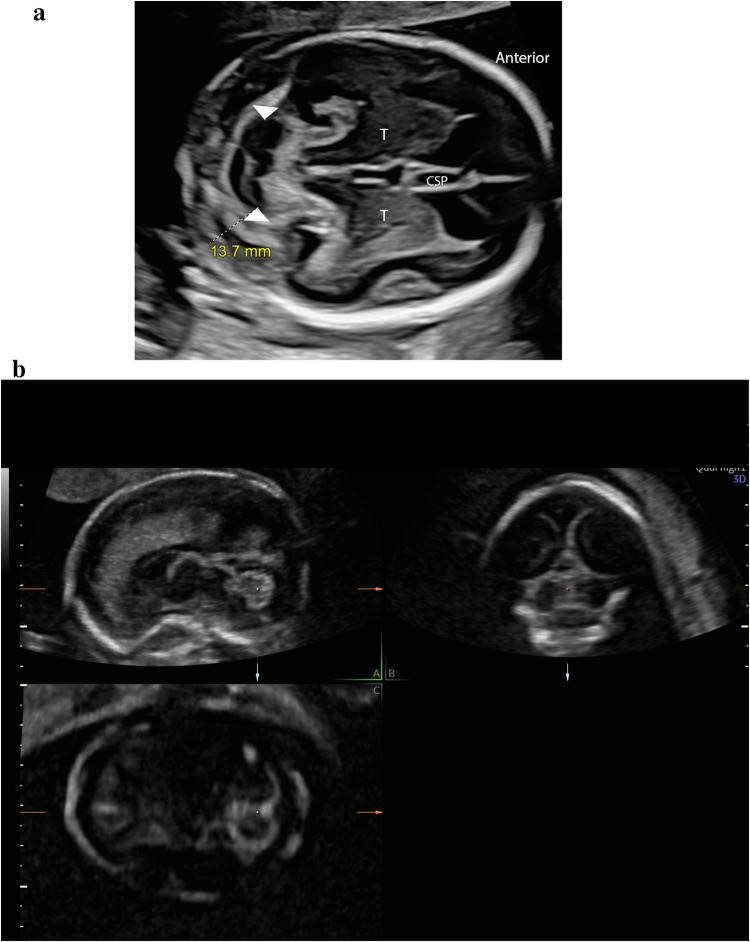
Ultrasound assessment of the posterior fossa in a fetus at 19 4/7 weeks. a Suboccipitobregmatic transverse view of the fetal head aligns the lower cavum septum pellucidum (CSP), thalamic nuclei (T), and cerebellum for accurate assessment of the transverse cerebellar diameter (TCD). TCD measures 13.7 mm (*between arrowheads*), which is less than the 1st percentile (50th percentile for TCD is 19.7 mm at this GA)^10^. b Three-dimensional ultrasound allows for the reconstruction of posterior fossa imaging to provide sagittal assessment of the vermis and craniocaudal measurement to improve the accuracy of diagnosis (*dot* denotes center of vermis in all three planes)
